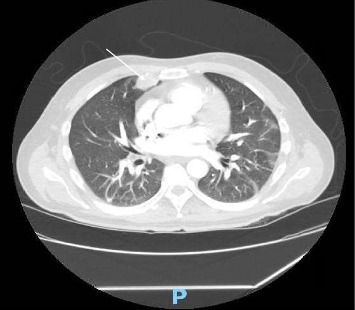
CT scans indicate that the cavitary lung lesions from previous scans have solidified and healed. (white arrow).
